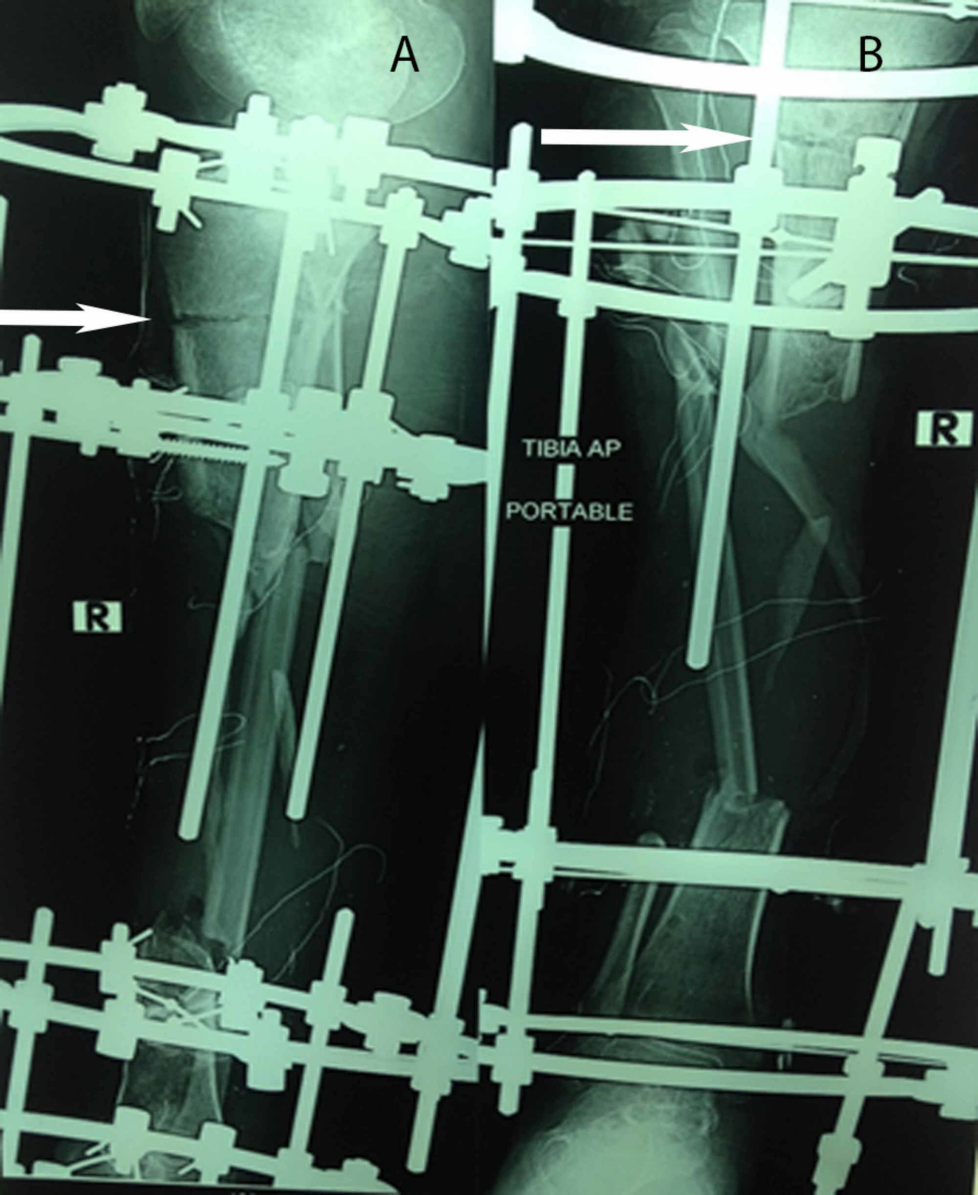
Immediate postoperative plain radiographs of the same patient who underwent multiple drill-hole osteotomy, with an anteroposterior (AP) view (2A) and a posteroanterior view (2B)
